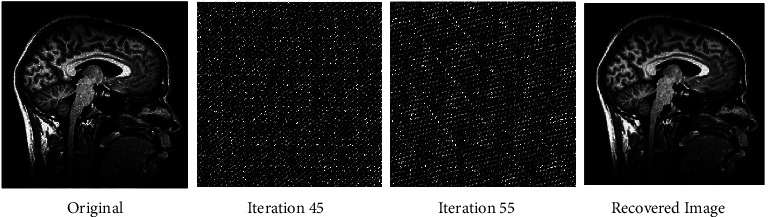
Before and after encryption of ACM (sagittal brain MRI).
